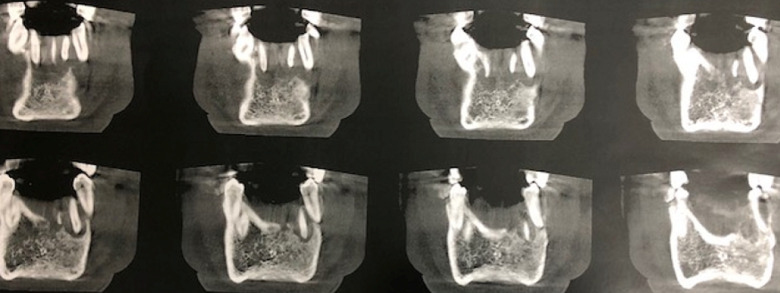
cone beam computed tomography, coronal slices
